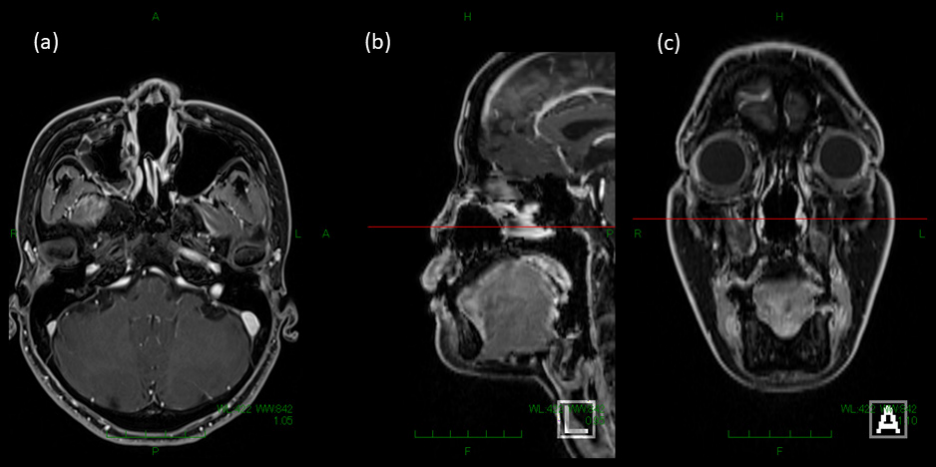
The figure shows magnetic resonance images taken 15 months after the completion of radiation therapy. (a), (b), and (c) represents the axial, sagittal, and coronal image, respectively. No obvious residual tumor was noted.
